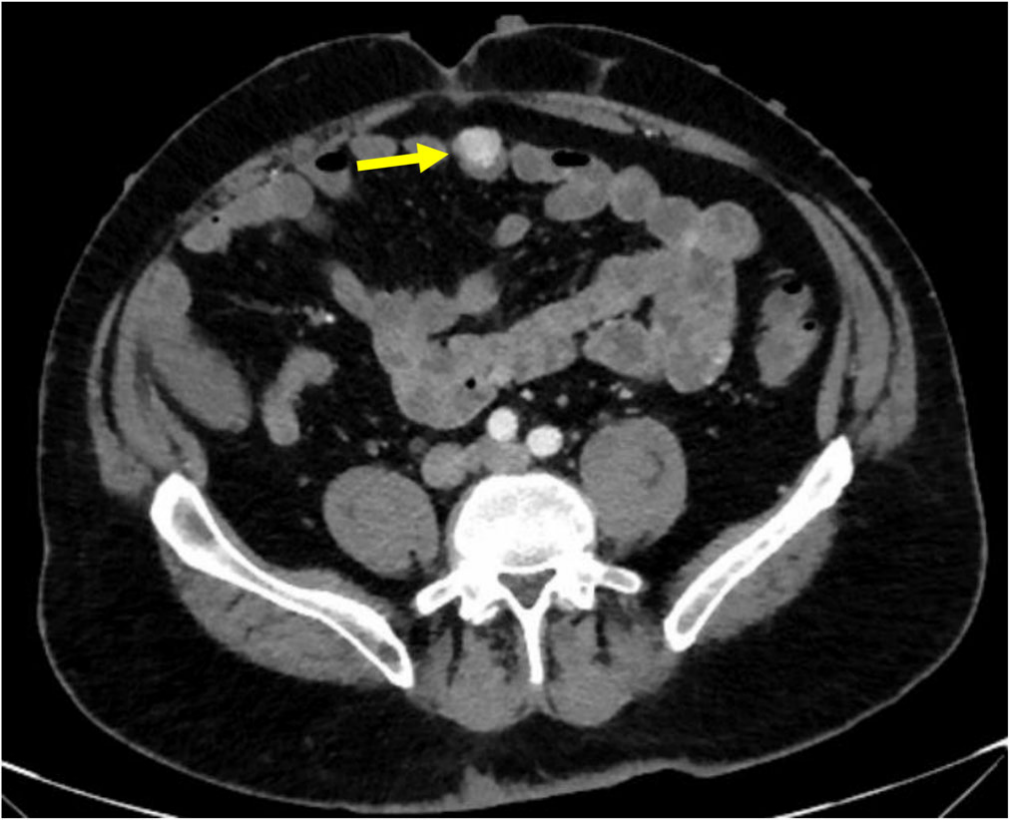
Axial slice CT abdomen in arterial phase demonstrating a hypervascular small bowel lesion in the mid‐abdomen (yellow arrow).
